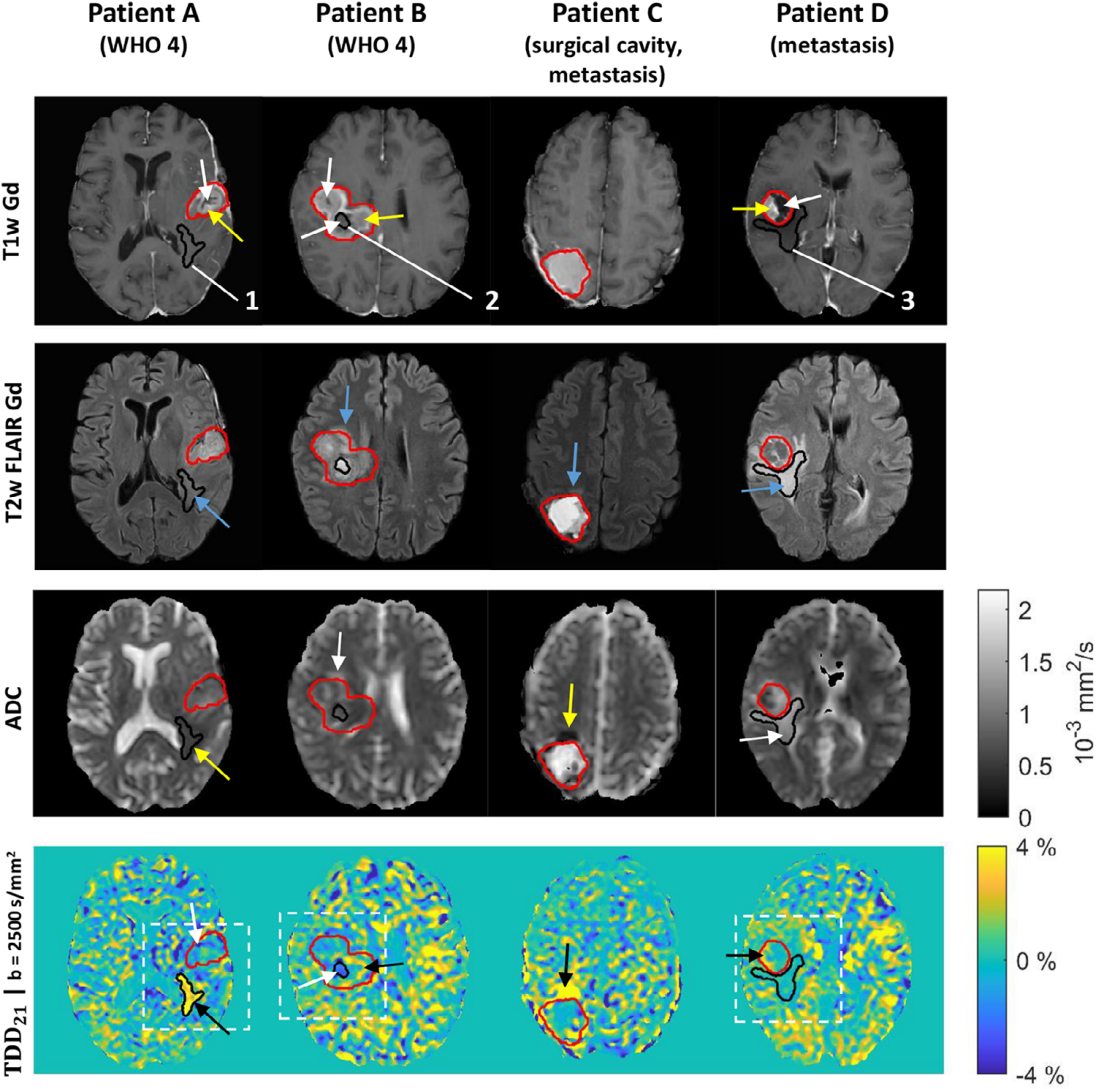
The first four patient cases. The GTV (red contour) and three example ROIs (black contours with labels 1–3) are shown on T1w Gd and T2w FLAIR Gd images, ADC map, and TDD_21_
